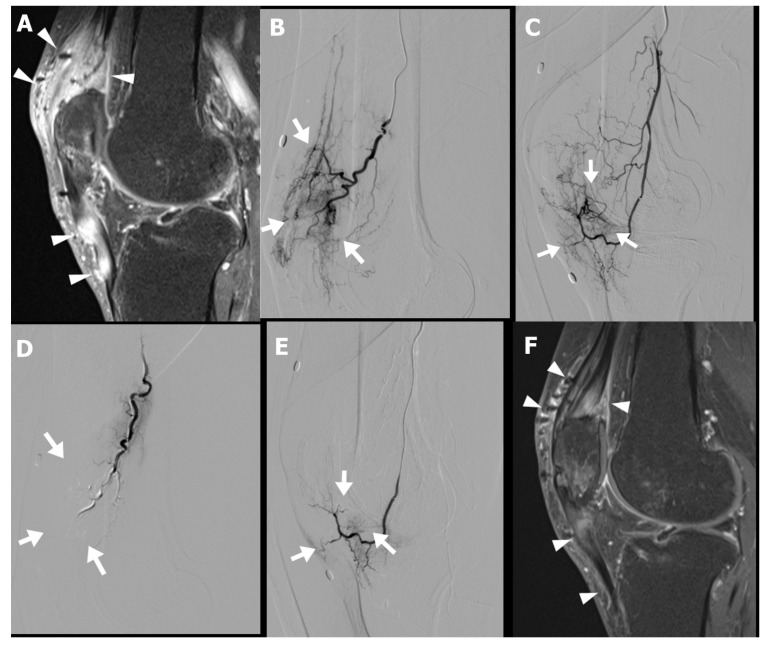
Preprocedural gadolinium-enhanced sagittal T1-weighted fat-suppressed magnetic resonance image (MRI) (A) of a 63-year-old male with chronic anterior knee pain persisting for 8 months following patellar fracture fixation demonstrates diffuse peritendinous and intratendinous contrast enhancement involving both the quadriceps and patellar tendons, as well as the adjacent soft tissue (arrowheads). Selective digital subtraction angiography of the superior patellar artery (lateral projection, (B)) and descending genicular artery (lateral projection, (C)) reveals abnormal angiogenesis (arrows in (B,C)) corresponding to the area of inflammation noted on MRI. Postembolization angiography (D,E) shows resolution of the abnormal vascular blush (arrows in (D,E)) indicating successful embolization of the pathological neovasculature. Follow-up MRI at 3 months postembolization demonstrates reduced peritendinous and intratendinous contrast enhancement (arrowheads in (F)), indicating decreased inflammation compared with the pretreatment MRI.
